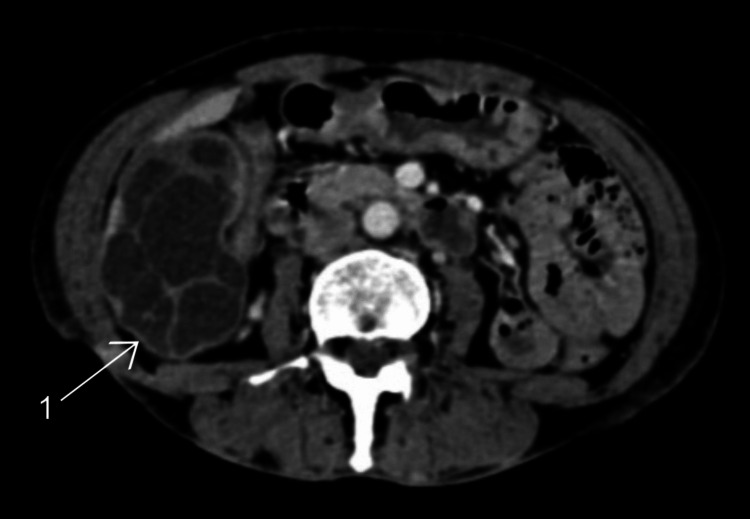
Computed tomography image of the abdomen. 1, hydatid cyst in the liver
